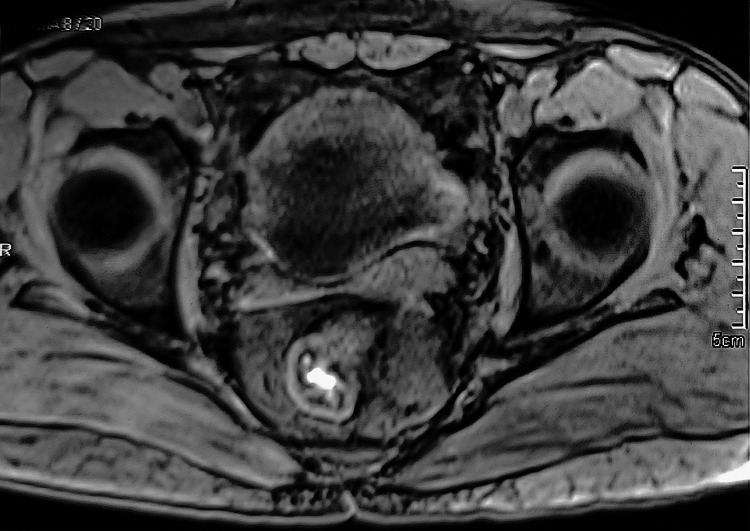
T2 weighted MRI image showing minimal residual disease
